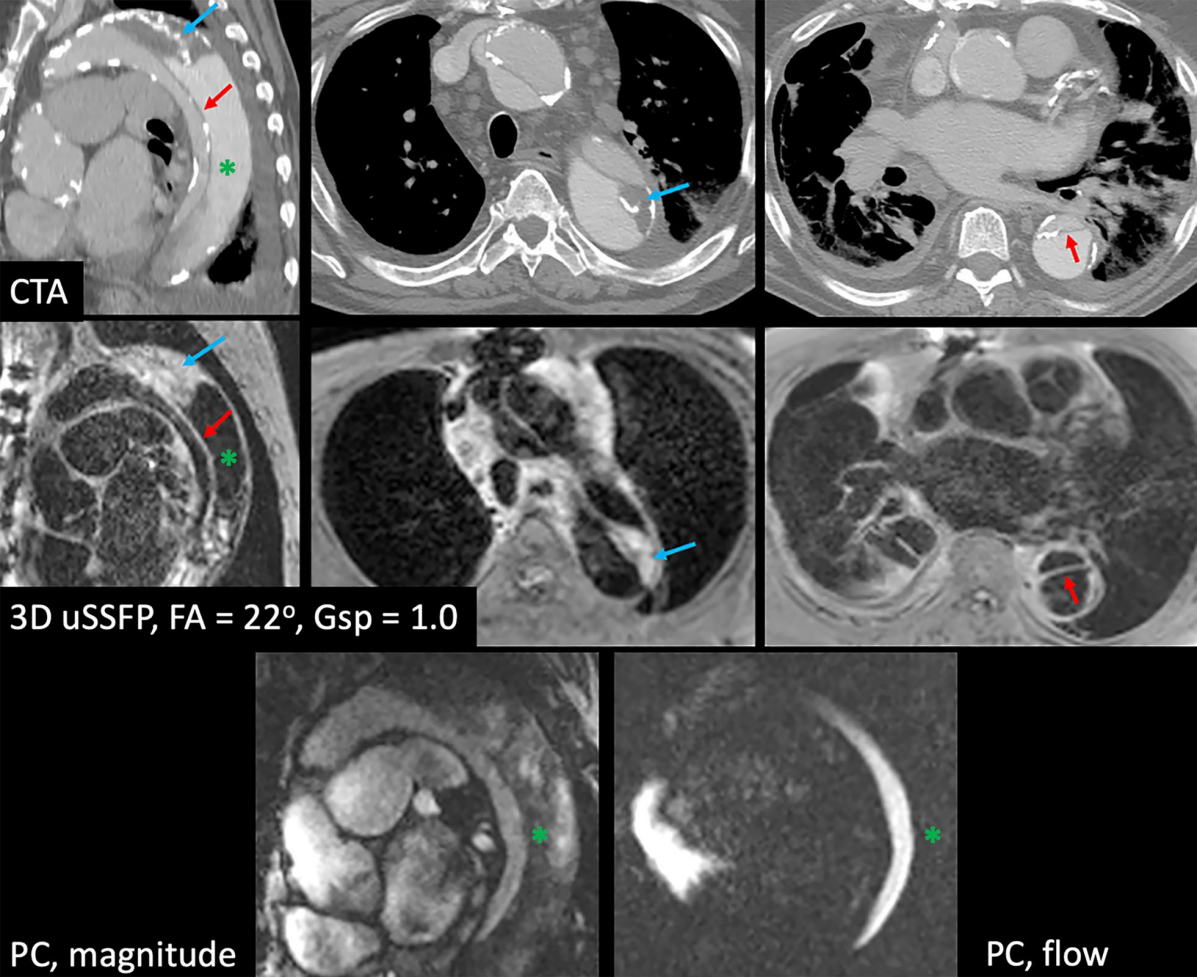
Repaired type A aortic dissection with very slow flow and thrombus (blue arrow) in the false lumen. *Top row* computed tomography angiography. *Middle row* 3D uSSFP. *Bottom row* Peak systolic magnitude (left) and flow (right) images from a phase contrast (PC) cine acquisition with in-plane velocity encoding sensitivity of 150 cm/s show negligible flow in the false lumen (*). Nonetheless, 3D uSSFP acquired with a gradient spoiler factor = 1.0 was able to suppress the blood pool signal in the false lumen, while the dissection flap (red arrow) is well demonstrated. Chronic intraluminal thrombus appears relatively bright with 3D uSSFP, as does mediastinal adenopathy
